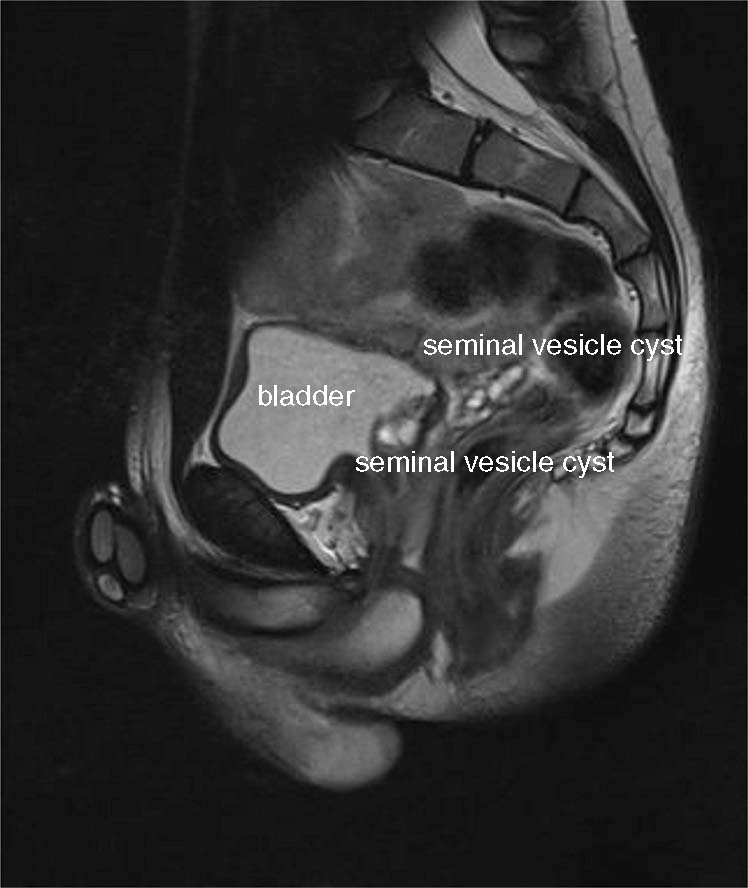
Preoperative MRI of the pelvis - demonstrating enlarged seminal vesicle cysts in the retrovesical space on the left-hand side.
